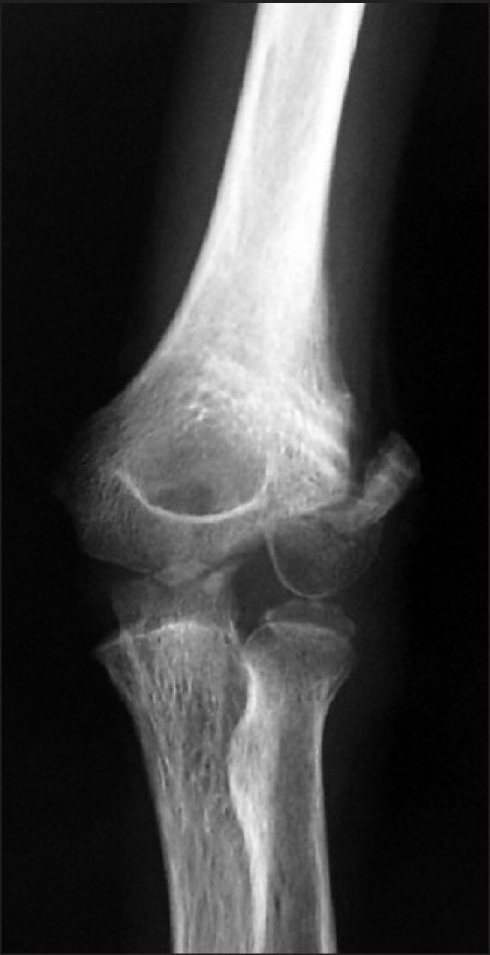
X-ray of the elbow joint (anteroposterior view) showing loss of reduction due to premature removal of K-wires
